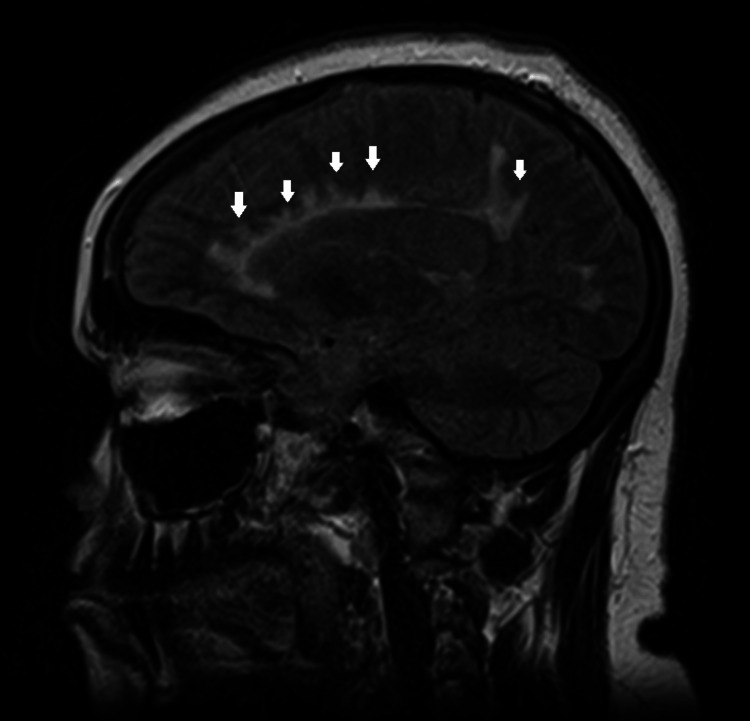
Sagittal MRI showing periventricular enhancement with radiations (white arrows) perpendicular to the body of the lateral ventricle (Dawson’s fingers). MRI: magnetic resonance imaging
